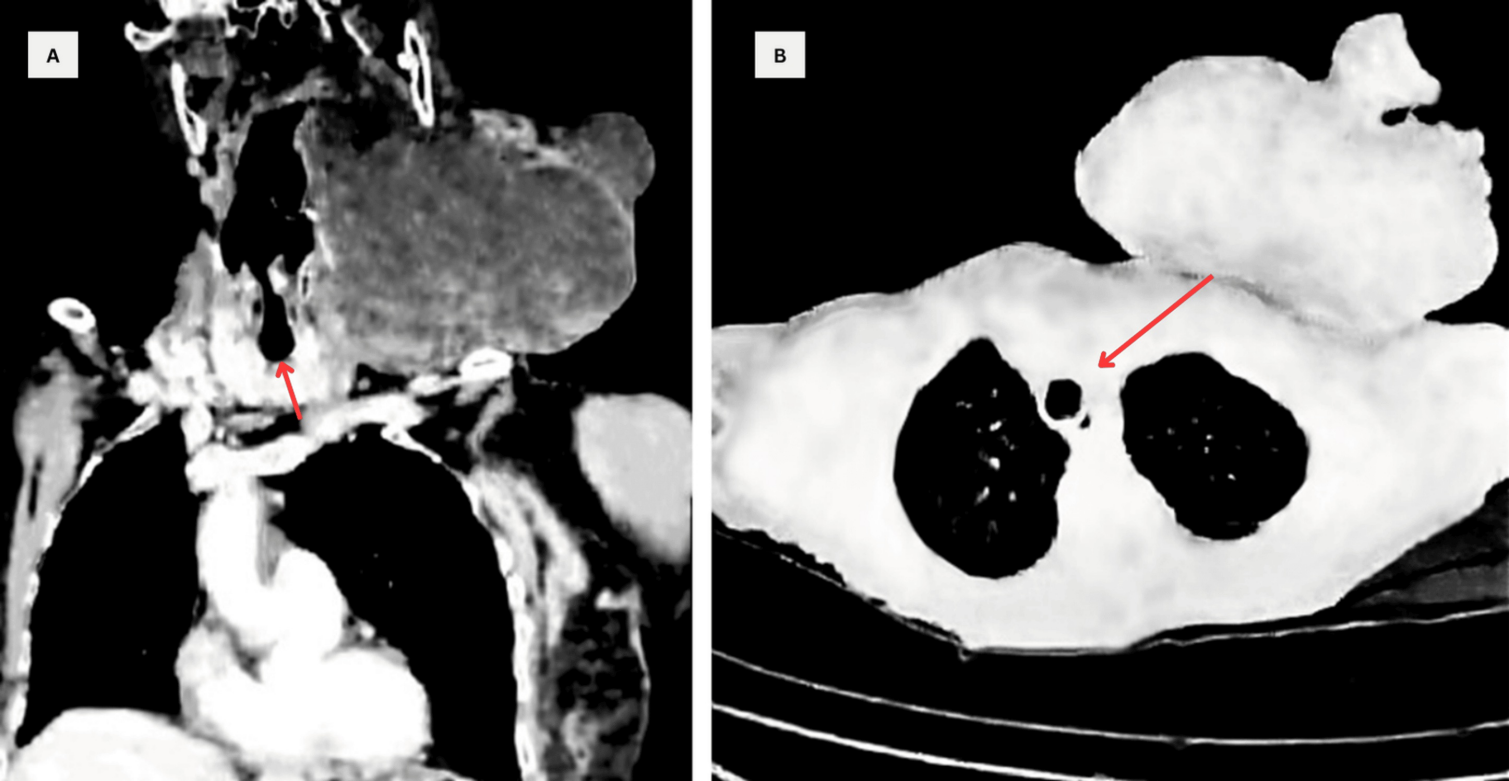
Contrast CT Images A: Coronal image showing a clearly demarcated, heterogeneous left cervical mass measuring approximately 30 x 25 cm, likely originating from the submandibular region, involving adjacent structures with no significant airway obstruction (red arrow). B: Axial images demonstrating tracheal deviation with a patent airway and no signs of obstruction (red arrow).
